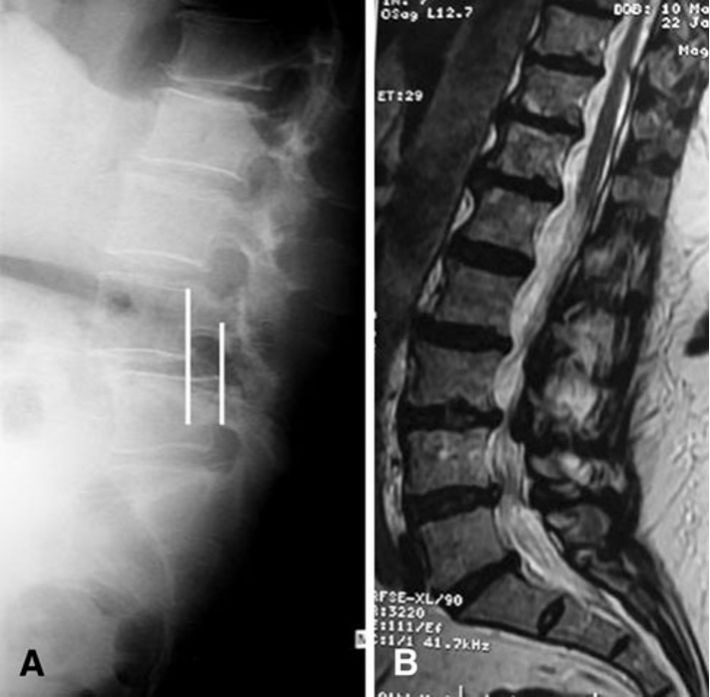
Lateral flexion radiograph of a 55 year-old male showing an L4 anterolisthesis of greater than 15% (a), which was unrecognized on the supine sagittal MRI (b)
